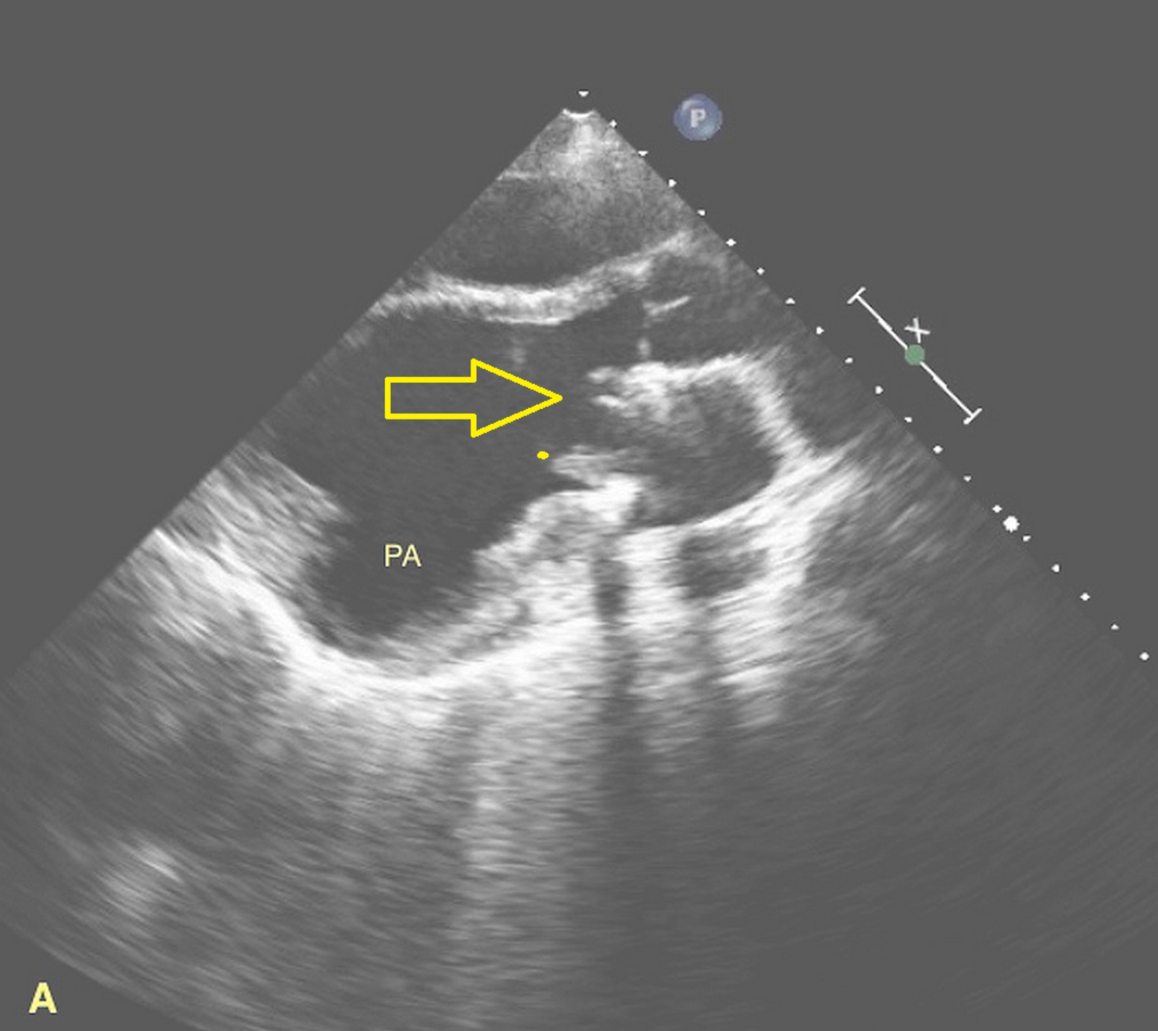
Two-dimensional echocardiography showing the left ventricular pseudoaneurysm (PA).
